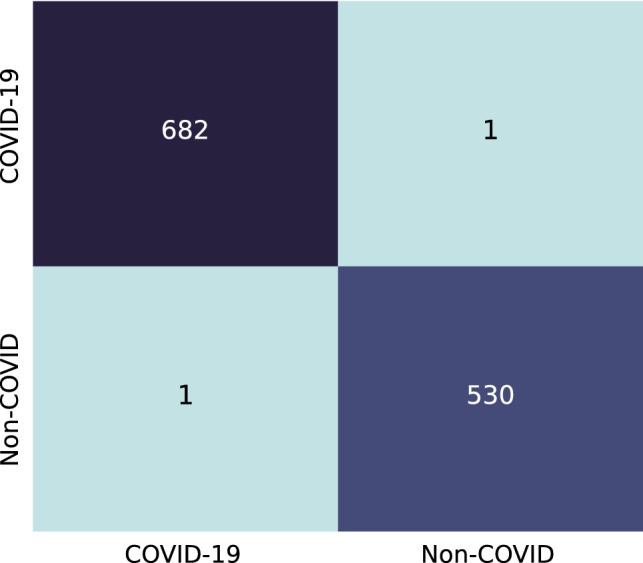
Confusion matrix for The IEEE COVID Chest X-ray Dataset
